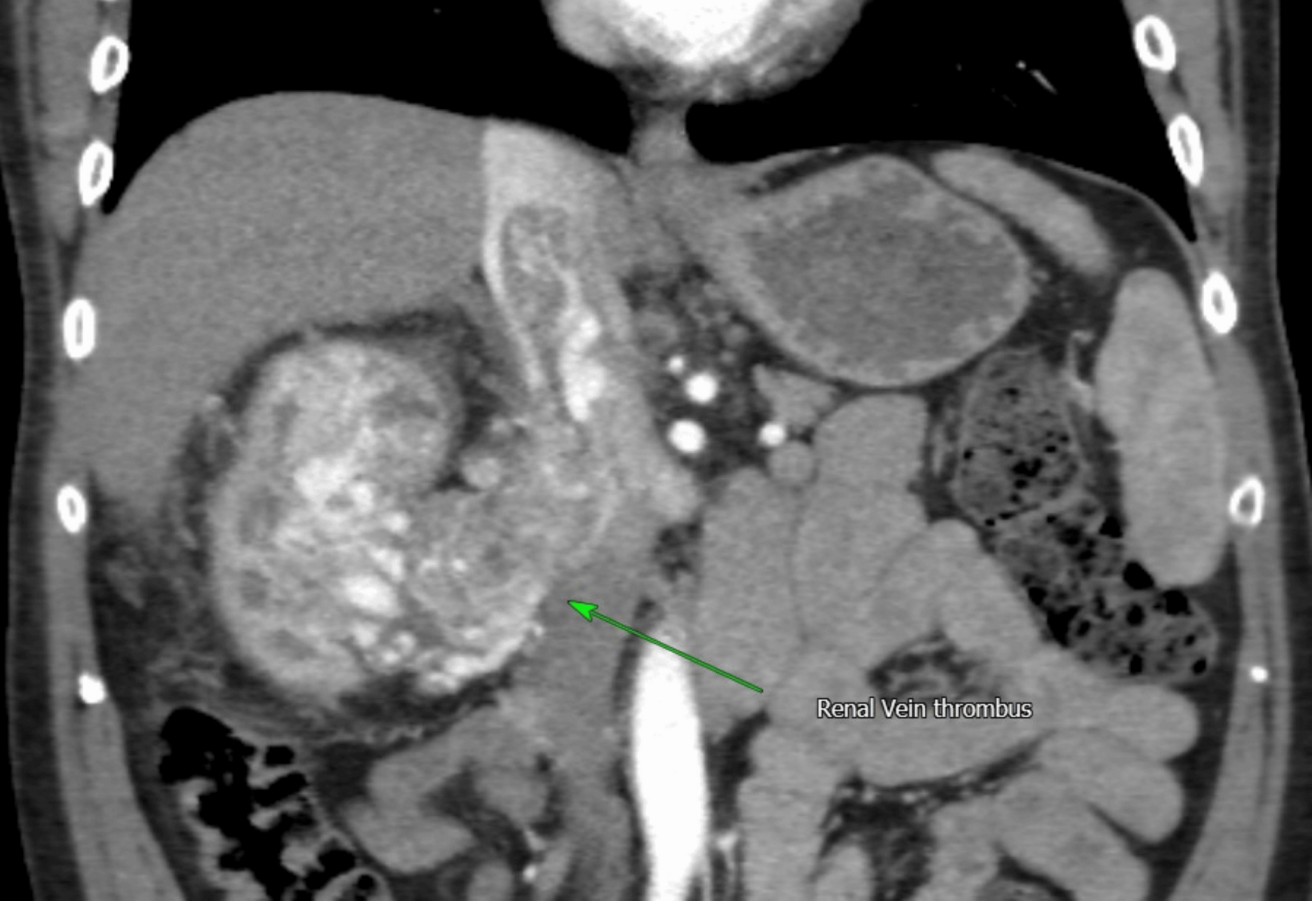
Coronal CT scan showing the level of extent of the IVC thrombus CT: computed tomography; IVC: inferior vena cava
